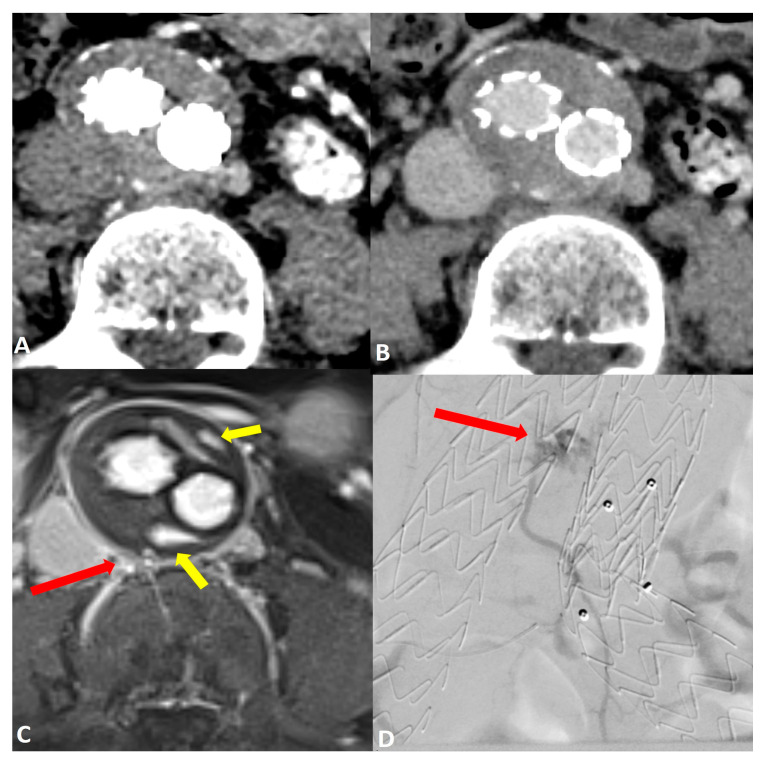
Post-operative imaging of a 57-year-old woman who underwent endovascular aneurysm repair (EVAR) of the abdominal aorta. Axial CT angiograms in (A) the post-contrast arterial phase and (B) the delayed phase did not reveal a clear endoleak within the growing aneurysm sac. Axial dynamic imaging utilizing the GRASP-VIBE sequence (C) revealed a type II endoleak with the right iliolumbar artery directly communicating with the aneurysm sac, denoted by a red arrow. Notably, there was an accumulation of contrast material at the anterior and posterior peripheral aspects of the aneurysm sac, marked by yellow arrows. This endoleak was subsequently confirmed in the digital subtraction angiography ((D), red arrow) and was promptly treated.
